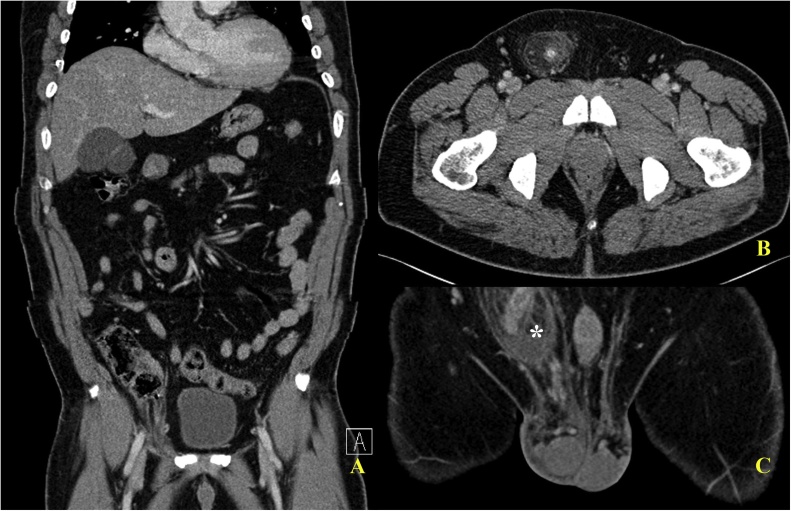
Contrast-enhanced CT images. (A) A blind-ended tubular structure incarcerated into the right inguinal canal (circle). (B) The incarcerated appendix contained an appendocolith. (arrow) (C) Inflamed appendix with perifocal stranding (asterisk).
